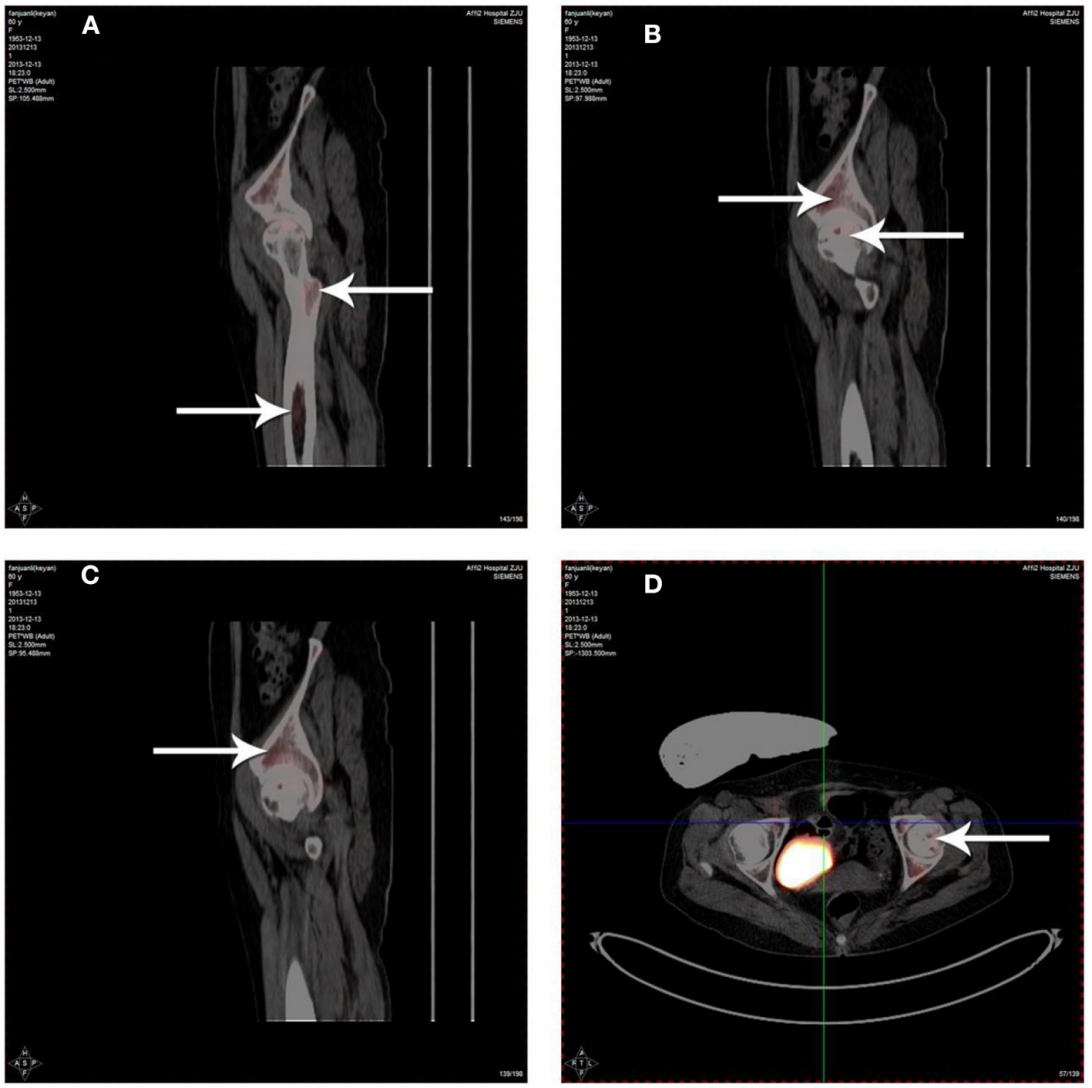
Distribution of ^18^F-FDG labeled PBSCs after infusion via the medial circumflex femoral artery. The ^18^F-FDG labeled PBSCs could be observed in the femoral bone marrow cavity (A), necrotic area of the femoral head (B,D) and acetabulum (C). The ^18^F-FDG labeled PBSCs could be seen in necrotic area of the femoral head indicating that PBSCs could enter into the femoral head and migrate into necrotic field of the femoral head participating in the repair of osteonecrosis after infusion through the medial circumflex femoral artery.
